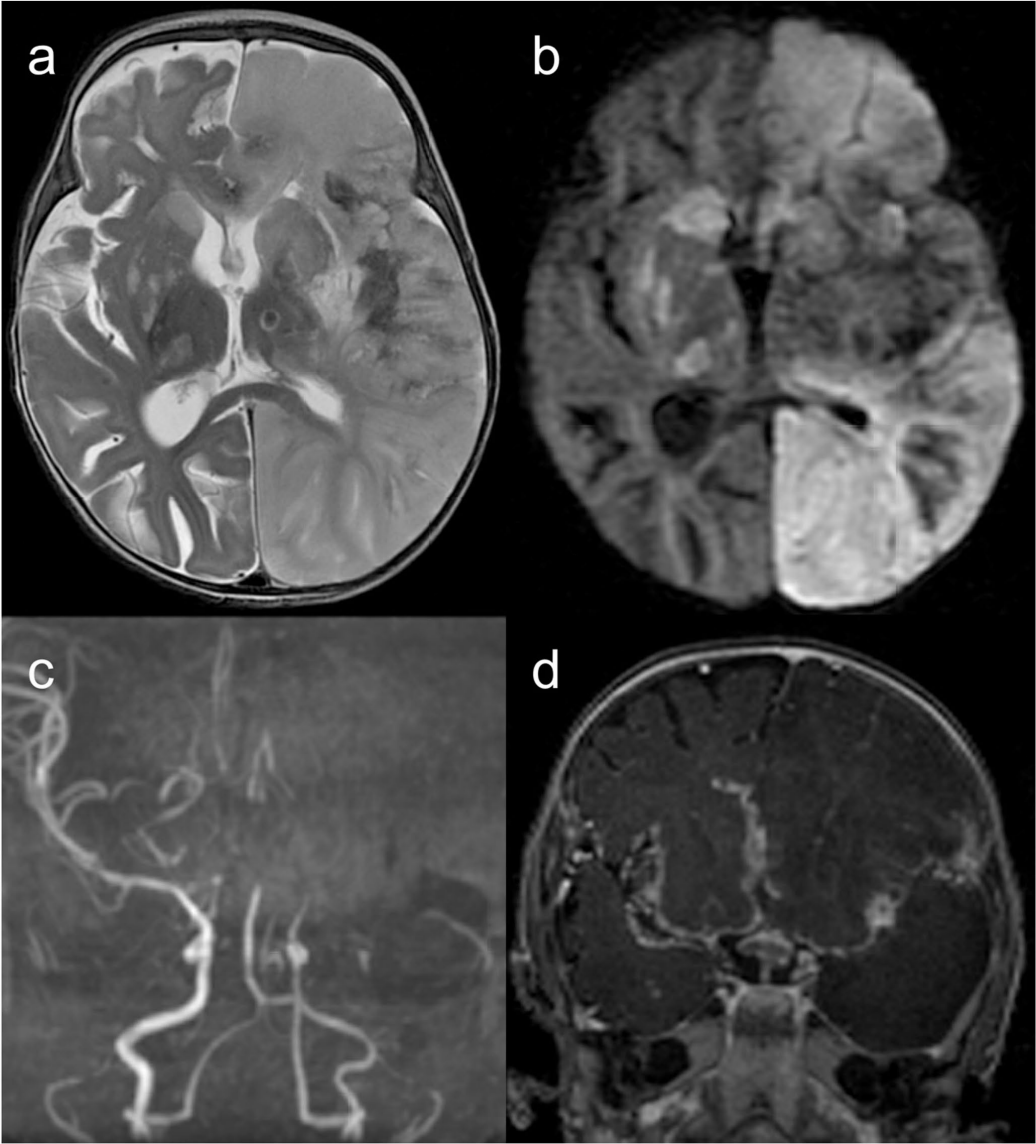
Immunodeficiency under investigation. A 6-month-old female patient with tuberculous meningitis complicated by ischemic stroke. Brain MRI (a – axial T2, b – axial b1000 DWI, c – coronal maximum intensity projection 3D TOF MR angiography, and d – gadolinium-enhanced coronal T1) reveals basal-predominant leptomeningeal enhancement and an acute infarction in the left cerebral hemisphere due to occlusion of the ipsilateral supraclinoid internal carotid artery. Additionally, there is hydrocephalus and bilateral acute ischemic lesions in the basal ganglia and thalami, attributed to small vessel vasculitis
